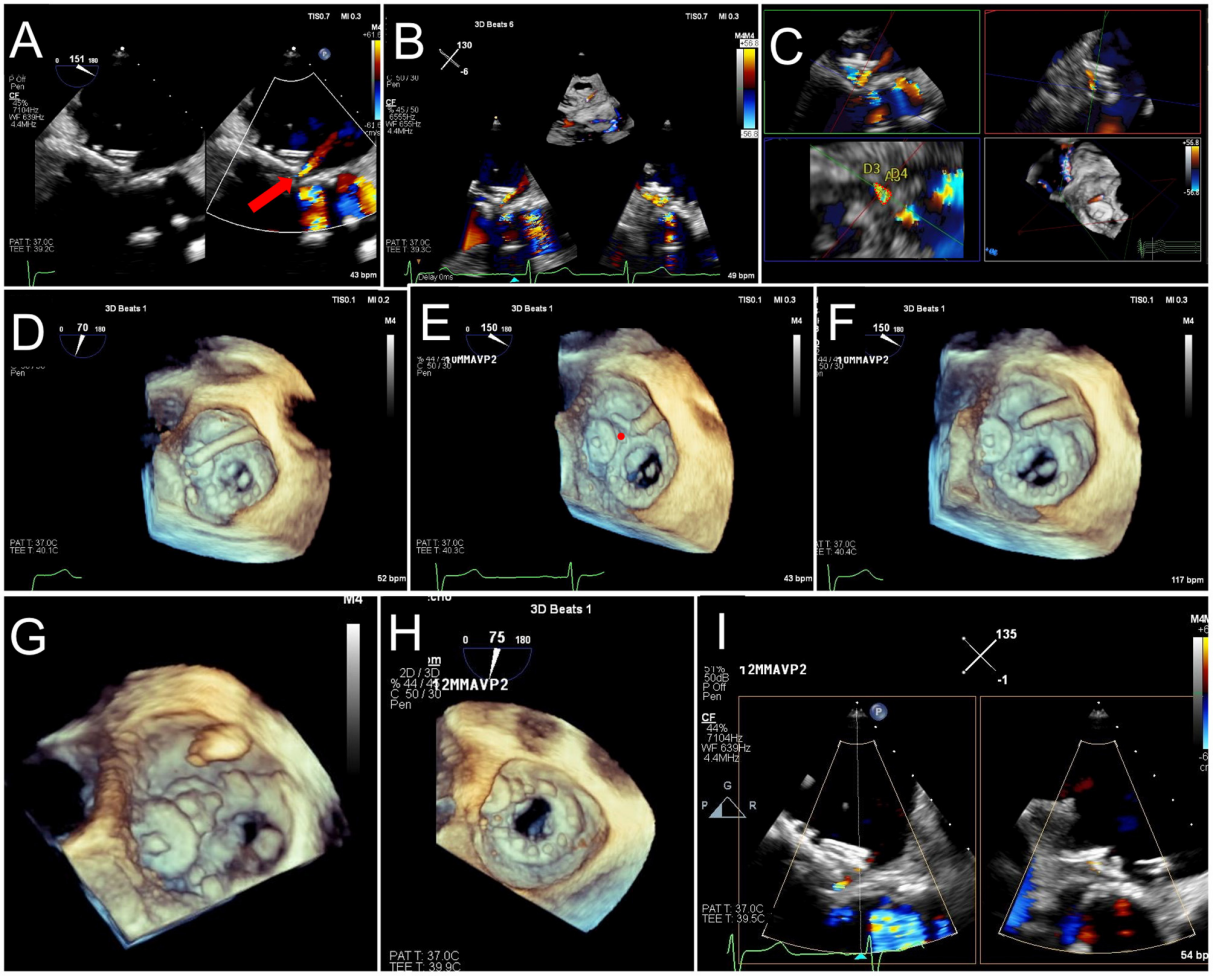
TEE Guided Implantation of a Second PVL Closure Device for Residual Leak. (A) After an initial deployment of a 10 mm AVPII device, a residual leak is seen adjacent to the first device (red arrow). (B) A sector-based 3D Color Doppler acquisition demonstrates the location of the residual leak medial to the first device. (C) Multiplanar reconstruction of the 3D color Doppler acquisition is aligned with the jet for measurement of the paravalvular defect (lower left panel, dotted red tracing), measuring 17 mm^2^, with dimensions 10 x 4 mm. (D) The guide catheter is positioned on top of the first device, too lateral to enter the defect. (E) The guide is pulled back, and wire crossing of the defect (red circle) is attempted. Wire trajectory is medial and anterior to the defect. (F) After correction of wire trajectory, the defect is crossed. (G) A 12 mm AVPII device is deployed within the residual defect. (H) Bioprosthetic leaflet opening appears normal, without interference from the closure devices. (I) Biplane color Doppler imaging demonstrates trivial residual leak after deployment of the 2nd PVL closure device.
